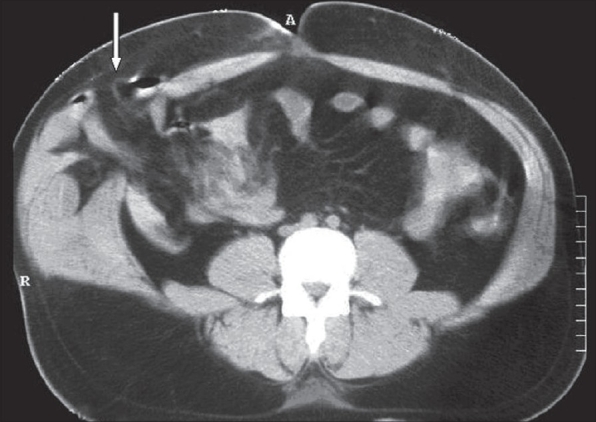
Axial CT of the abdomen: A defect at the anterior abdominal wall muscles (arrow) with bowel loops herniating through it
